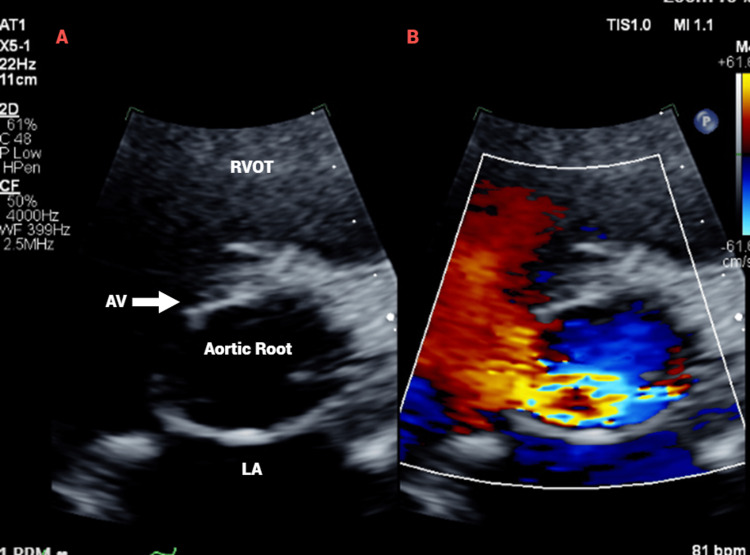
Transthoracic echocardiogram: Parasternal short-axis (PSAX) view at the level of the aortic valve (AV). (A) showing structures at the level of the AV. (B) showing structures with added colour Doppler consistent with moderate aortic regurgitation; no vegetations were noted. Left ventricular internal dimension at end-diastole (LVIDd) was 4.6 cm, and left ventricular ejection fraction (LVEF) was estimated at 55-60%. Vena contracta width measured 5 mm, with no holodiastolic flow reversal in the descending aorta. LA: left atrium; RVOT: right ventricular outflow tract
